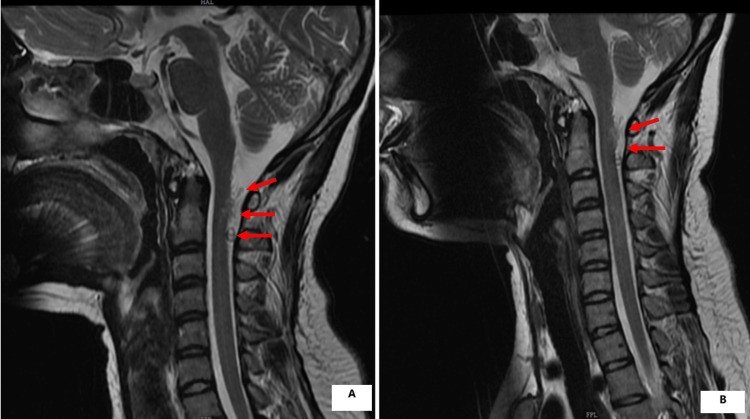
(A) Preoperative MRI of the patient. (B) Postoperative one-year follow-up MRI of the patient MRI: magnetic resonance imaging
